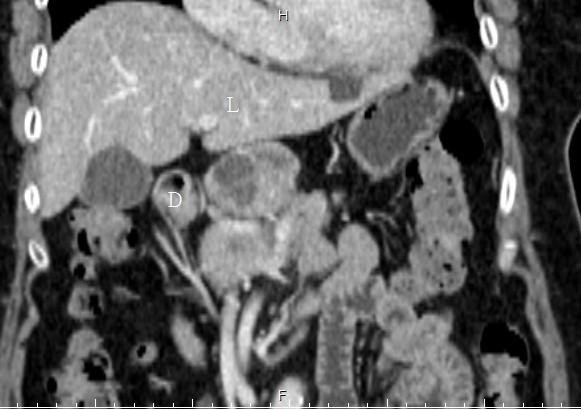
Computed tomography findings. A mass was between the duodenum (D) and liver (L).
